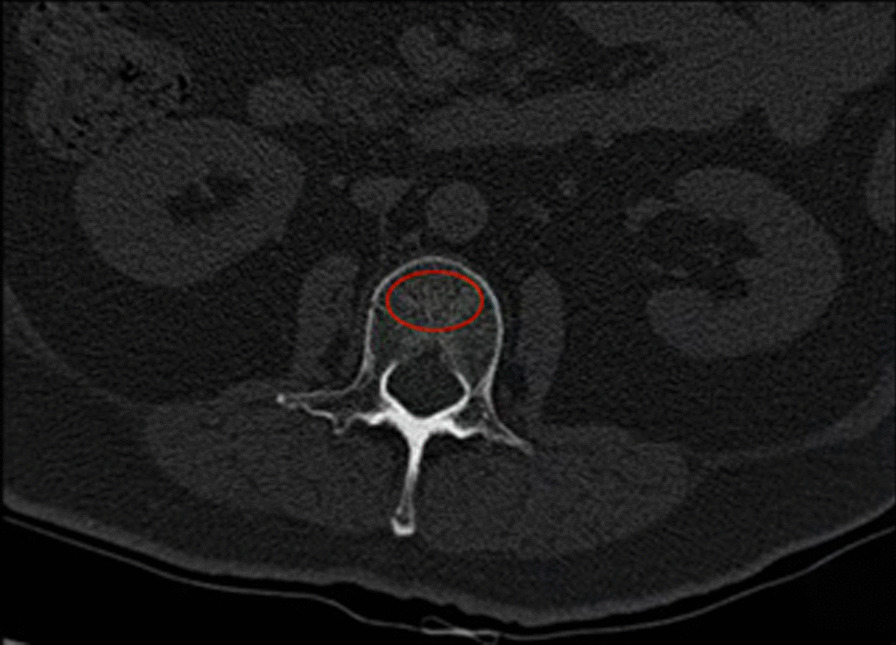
Place an as-large-as-possible oval ROI in the cancellous tissue of the vertebral body, and read out the average CT value of this area directly to indicate the bone mineral density of the patient
